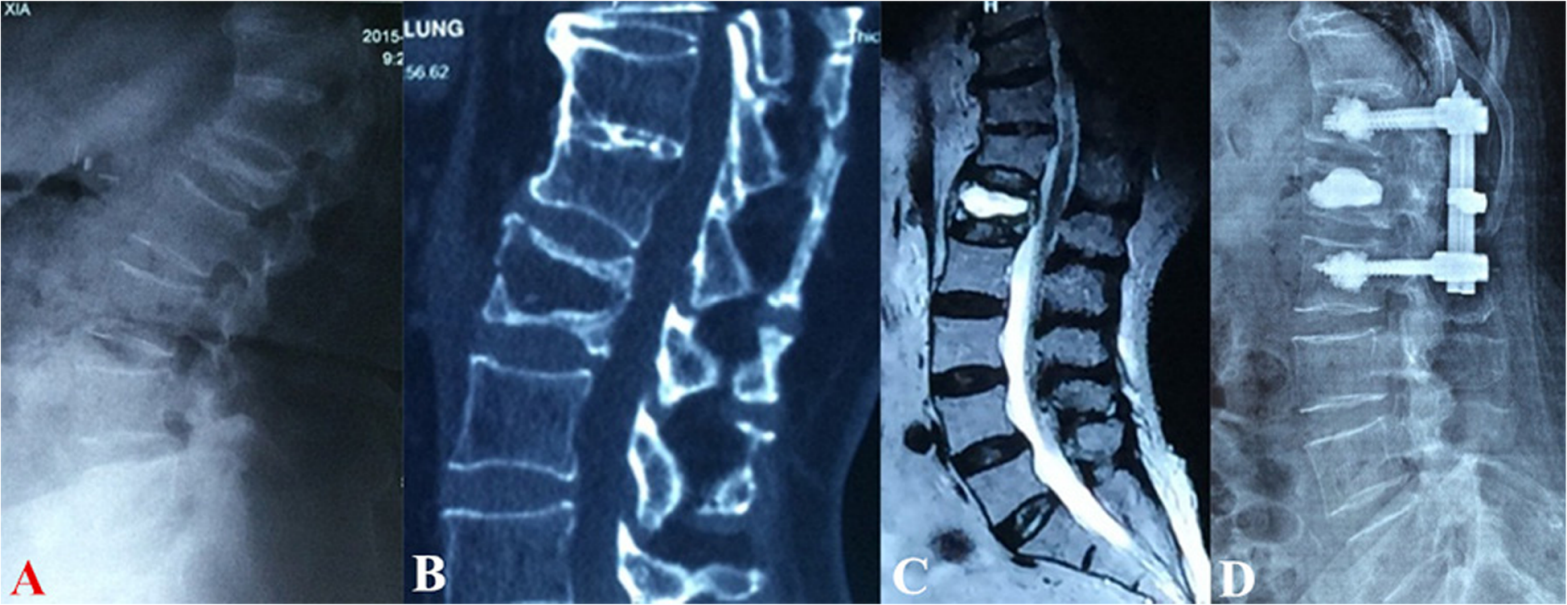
Type II treated using vertebroplasty with short-segment pedicle screw fixation and posterolateral fusion. a–c The preoperative flexion lateral radiography, supine CT reconstruction view, and magnetic resonance imaging respectively. d Postoperative lateral radiography
